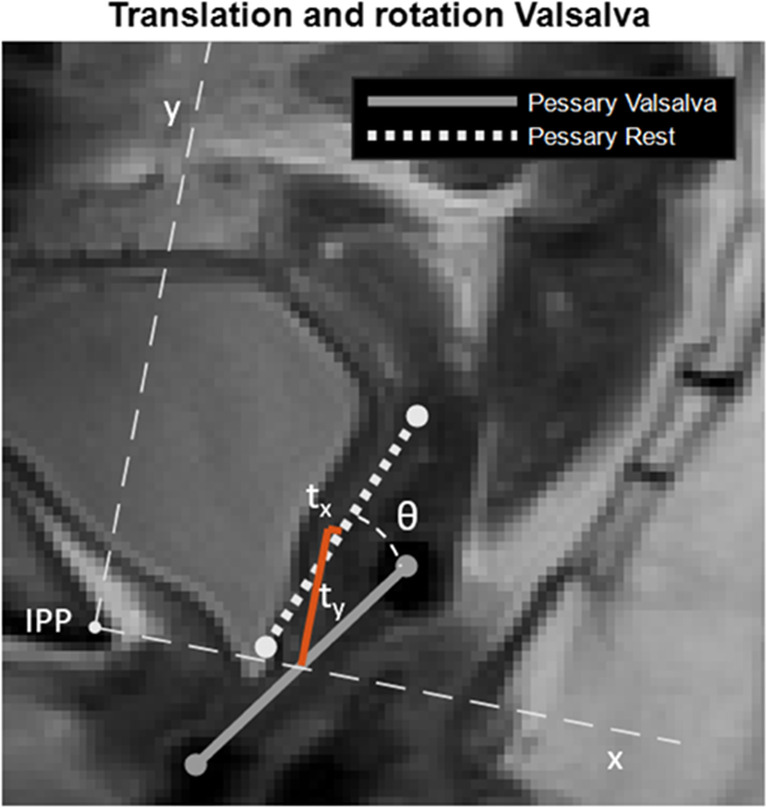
Landmarks on dynamic magnetic resonance imaging showing pessary rotation and translation in x- and y-directions. The *dashed white line* represents the pessary at rest and the *solid grey* at Valsalva (line through the pessary cross-sectional points). The origin is located at the inferior pubic point IPP. The x-axis points posteriorly and the y-axis cranially. The definition of pessary translation in x- and y-directions is shown with t_x_ and t_y_ respectively represented by the *red line*. Pessary rotation from rest to Valsalva is denoted by *θ*
